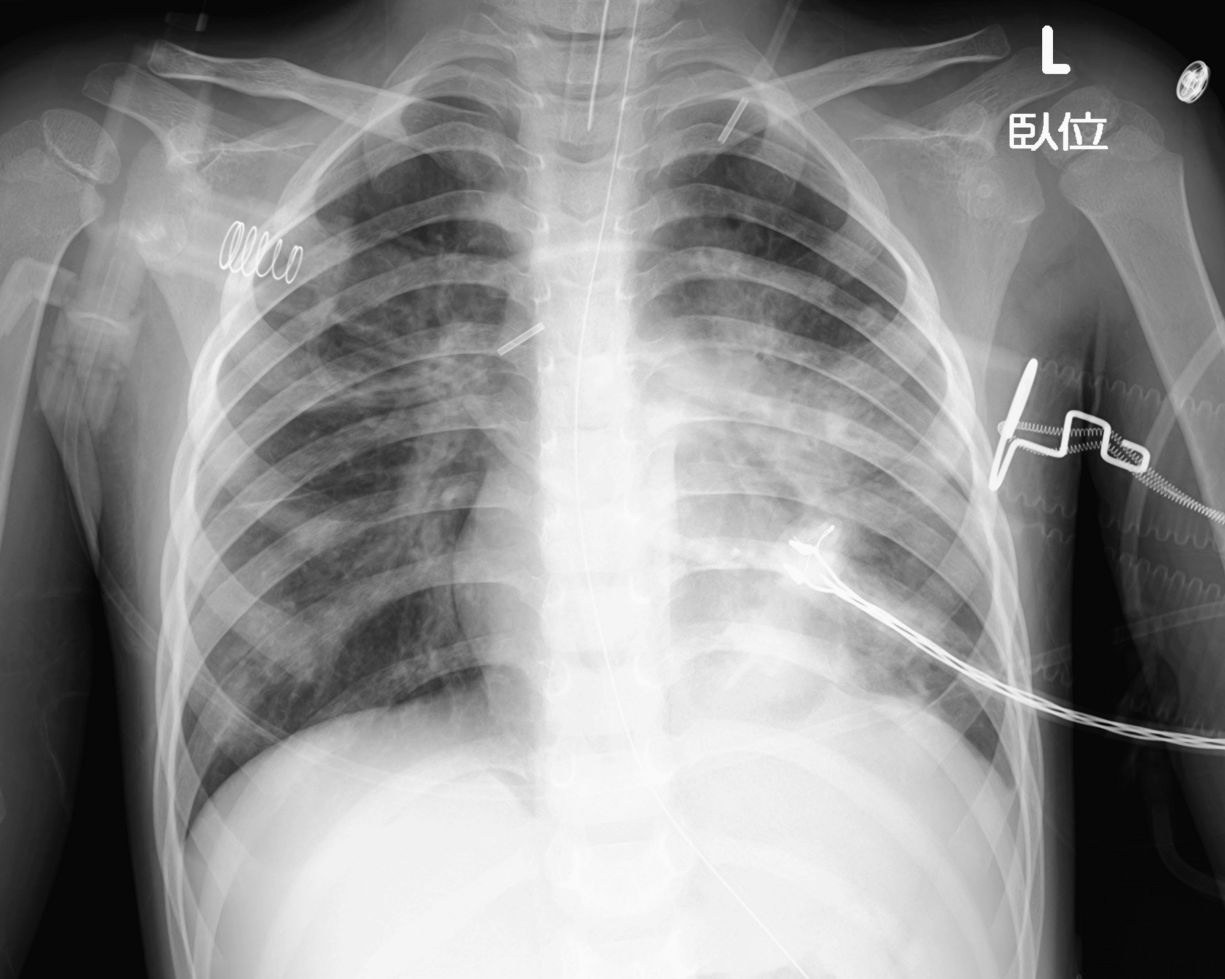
Chest X-ray after bronchoscopy The chest X-ray after removal of the mucus plug via bronchoscopy showed improvement in the atelectasis of the left lung.
